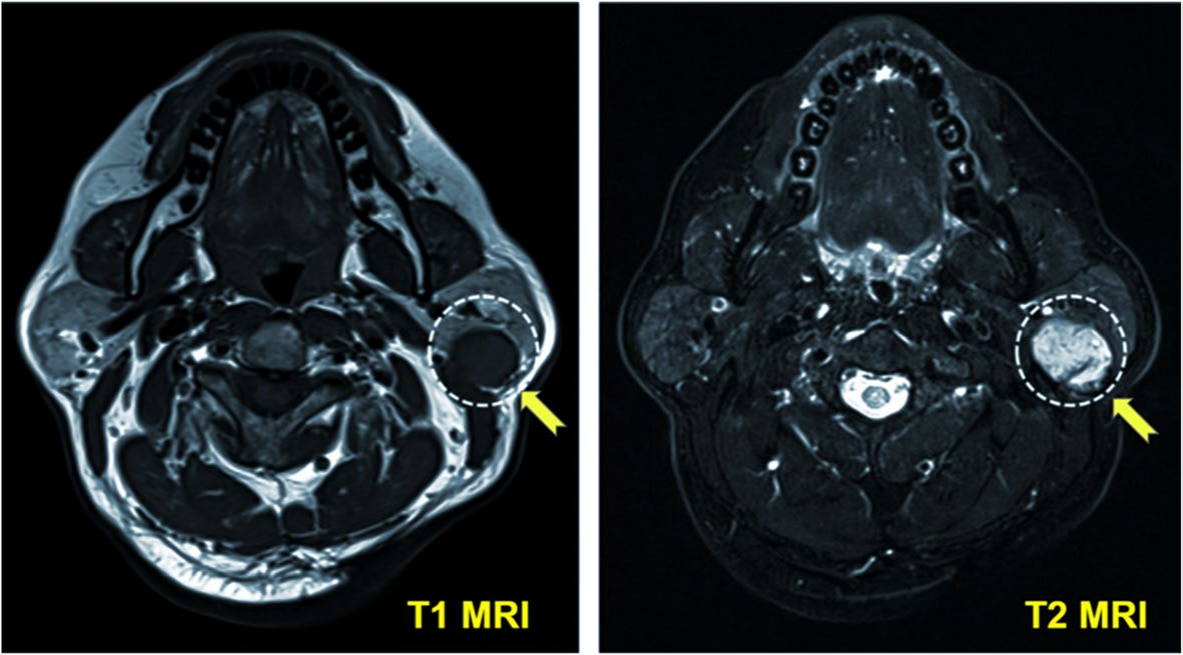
Example of using the ViT for tumor classification in MRI images using TransMed [53]. The tumor is enclosed by the dashed circle indicated by the yellow arrow
